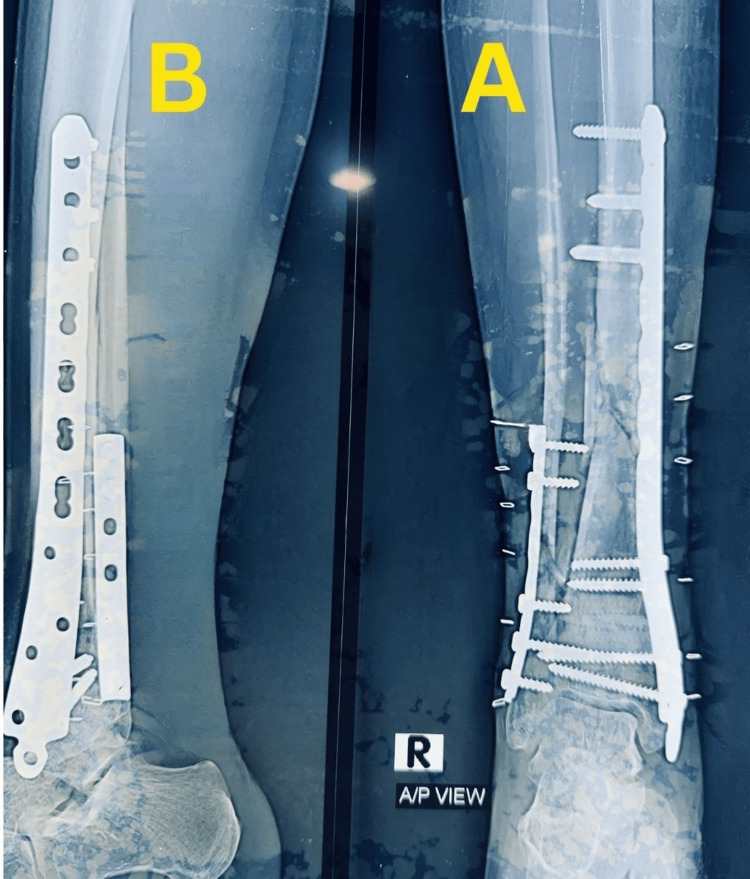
Immediate postoperative radiographs of Case 3. (A) Anteroposterior view showing fixation with a medial distal tibial locking plate and fibular plate with satisfactory alignment. (B) Lateral view confirming stable fixation and restoration of alignment.
